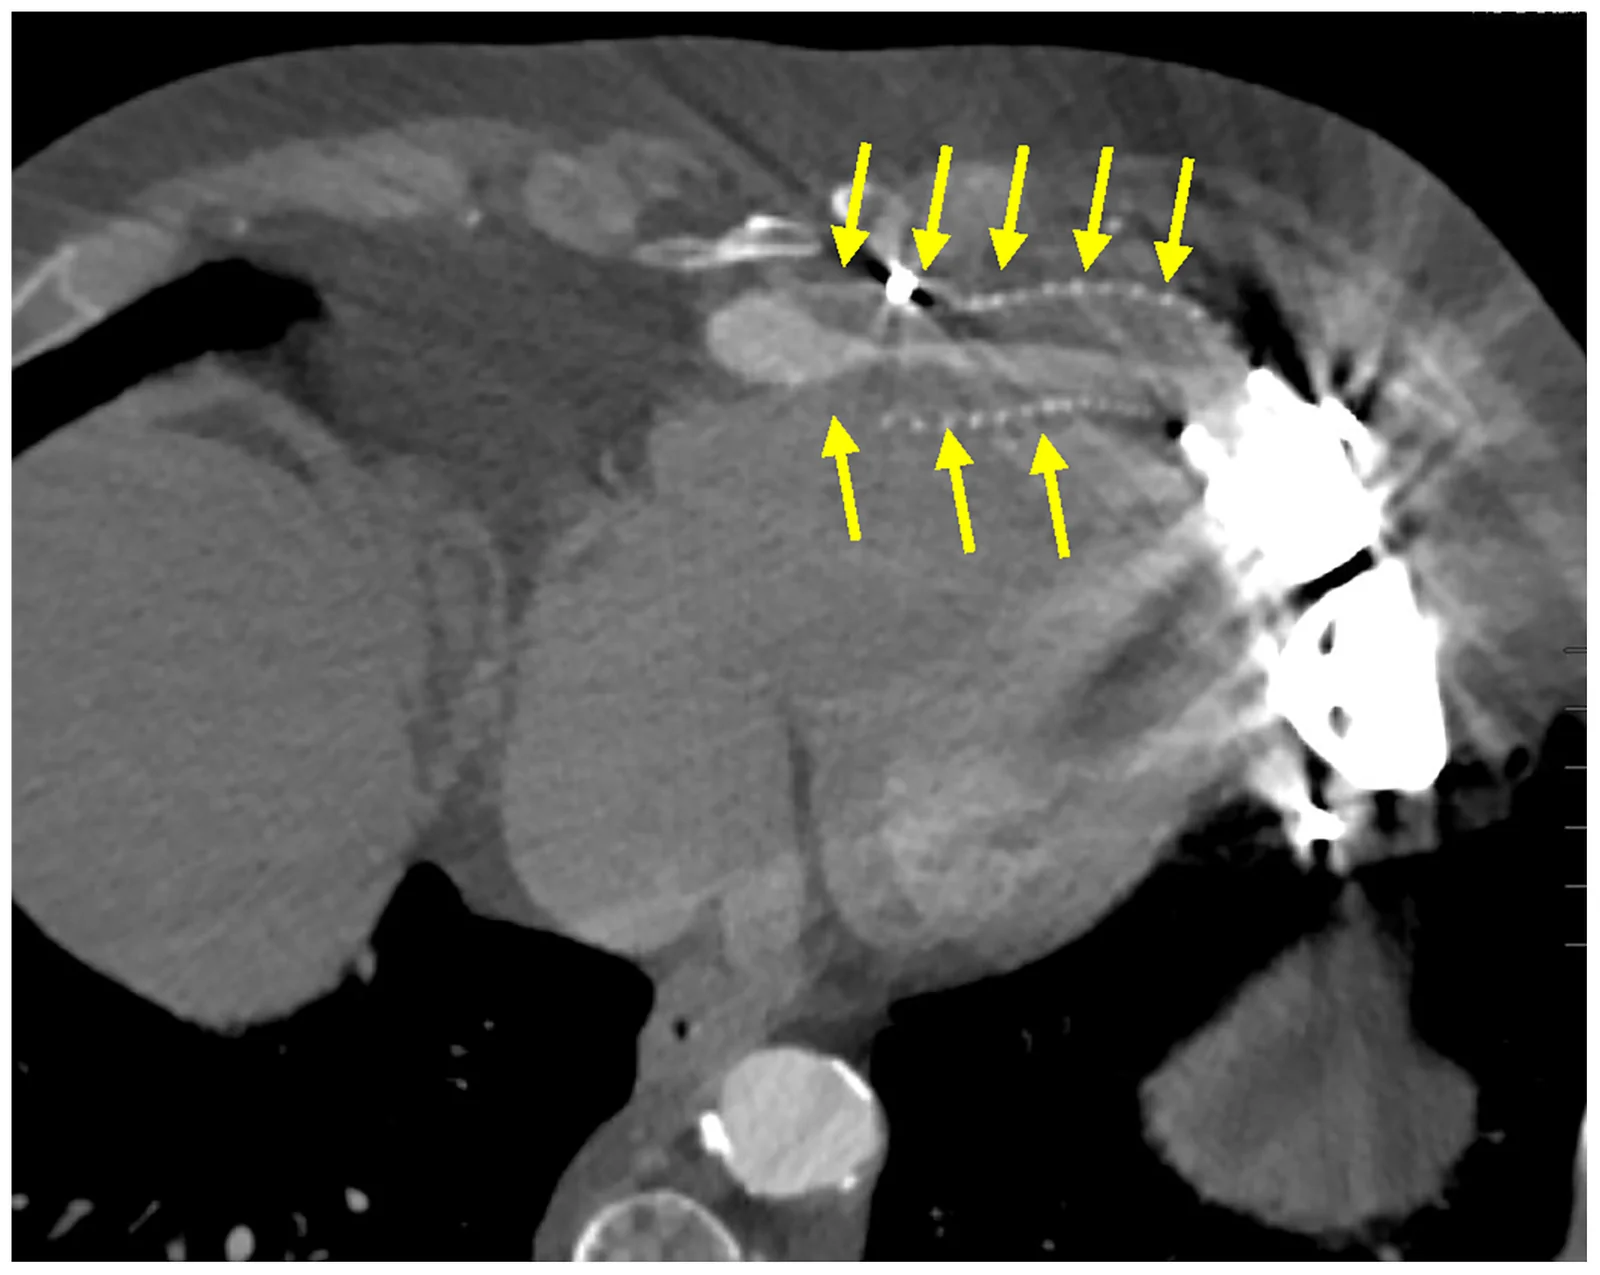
Preprocedural computed tomography angiography (CTA) demonstrating long-segment thrombosis and high-grade stenosis (*yellow arrows*) of the left ventricular assist device (LVAD) outflow graft within the bend-relief segment.
